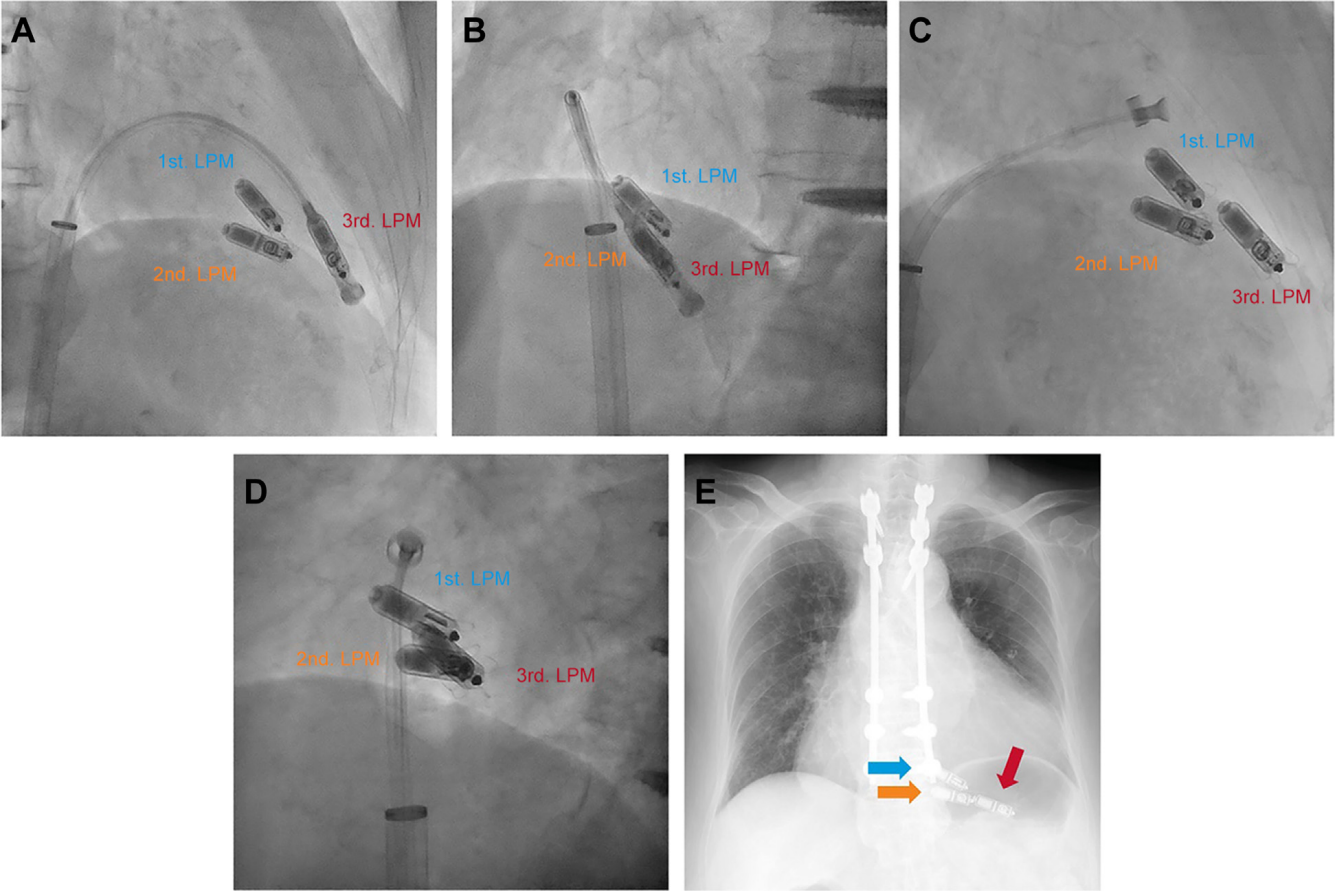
Third LPM Implantation Imaging (A and B) before and (C and D) after cutting the tether at 30° right and 50° left anterior positions. (E) Chest radiography showing the first, second, and third leadless pacemaker (LPMs), indicated by blue, orange, and red arrows, respectively.
